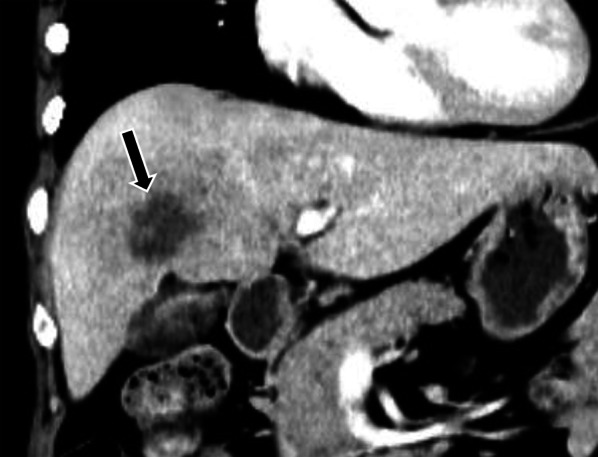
Typical mass-forming appearance of metastatic ILC in the liver in a 62-year-old female. She had Stage 1B ILC treated 4 years ago and was on adjuvant exemestane. Coronal contrast-enhanced CT image in the liver window shows a dominant hypodense mass in the liver (arrow) along with multiple other hepatic nodules (not pictured). Percutaneous biopsy of the liver mass showed metastatic ILC
